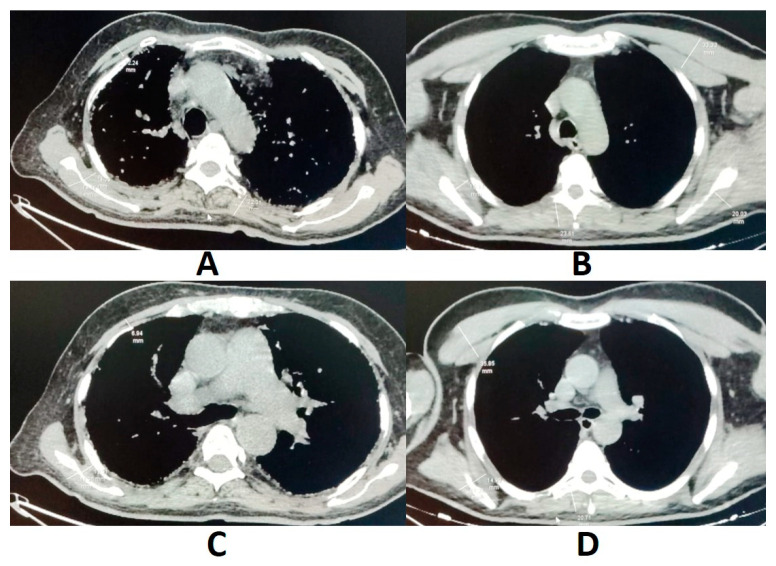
Representative CT slices showing chest wall muscle thickness measurements in IPF patients and controls. Muscle thickness was measured on axial CT images. Arrows indicate measurement points: (A,B) at the level of the aortic arch; (C,D) at the level of the pulmonary trunk. Abbreviations; IPF, idiopathic pulmonary fibrosis; CT, computed tomography.
